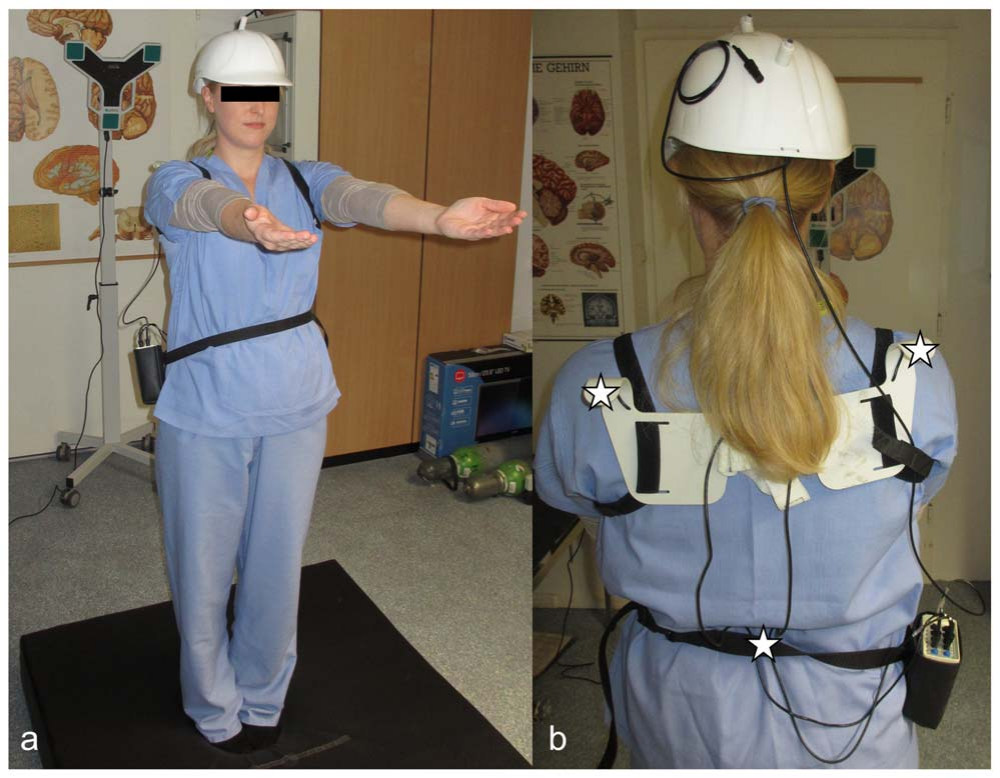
Test arrangement. (a) Body posture during Romberg's test for sway path evaluation with the feet close together, arms straight forward, palms facing up, and eyes closed (top). 20-cm-thick foam cushion used to minimize proprioceptive feedback during sway path evaluation (bottom). (b) Three ultrasound transmitters (*) positioned on the two shoulders and the lumbar spine were used for data generation.
